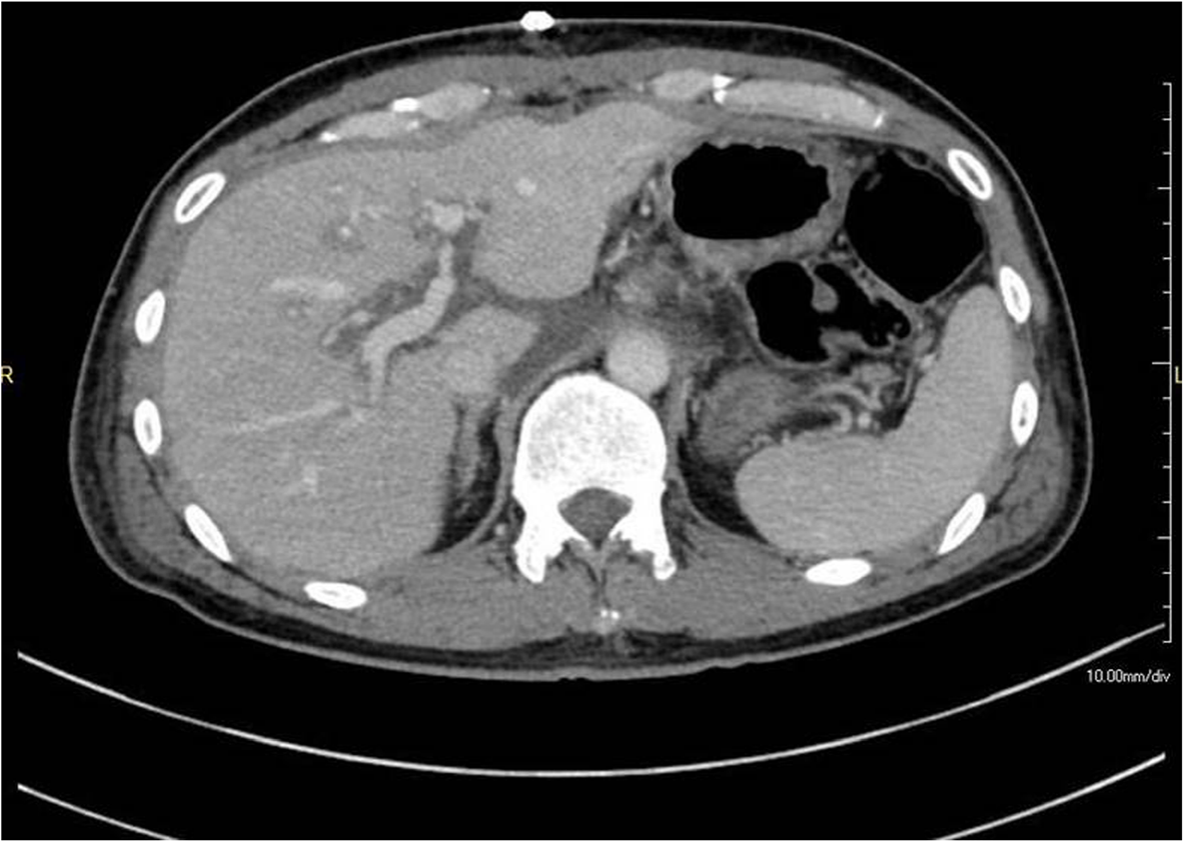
CT scan 11 days after reoperation showed that the area of liver ischemia was reduced
